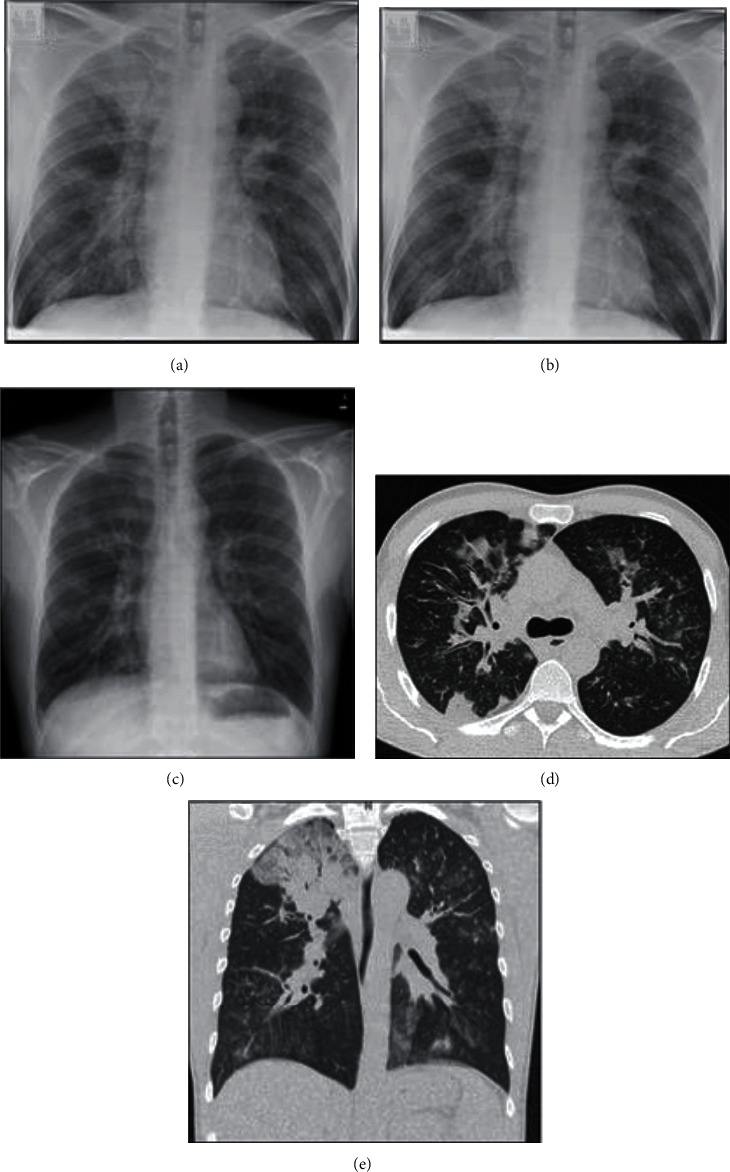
(a) Chest radiograph on the day of admission (March 14, 2020) to an external hospital shows consolidation predominantly in the right upper lobe, vague ill-defined opacities in the right lower lobe and left hilar region, and a diffuse interstitial pattern combined with bronchial wall thickening. (b) Chest X-ray on the day of admission to a tertiary care hospital depicts progressive pneumonia characterized by diffuse reticular and nodular patterns (March 22). (c) Chest X-ray shortly after discharge from the ICU (April 2) shows almost complete regression of previous infiltrations. The patient did not need supplementary oxygen at that time. (d, e) Computed tomography of the chest on March 16^th^ confirms consolidation of the right upper lobe and reveals multifocal, patchy consolidations, ill-defined airspace infiltrates, and ground-glass opacifications. Additional centrilobular nodular appearance and thickening of the bronchovascular structures are present.
